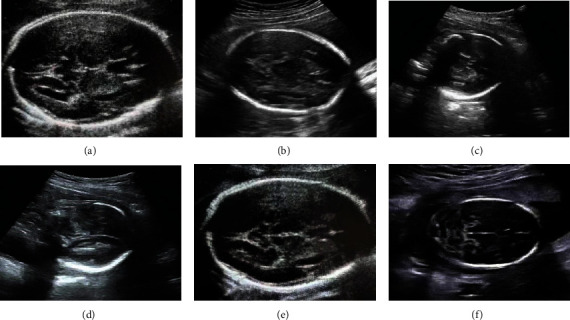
Color Doppler ultrasonography image.
